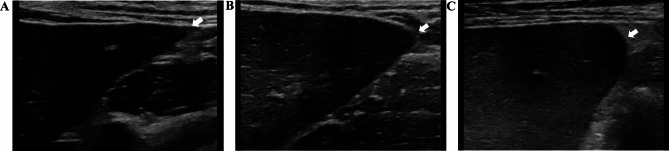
The ultrasound findings of the liver edge. (A) Sharp; (B) mildly dulled; (C) dulled
